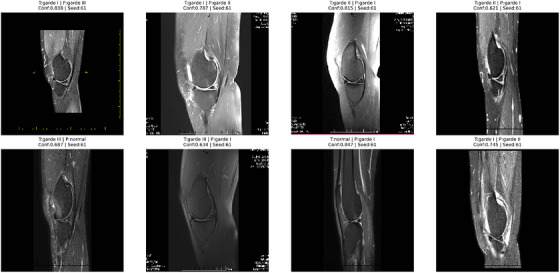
Representative misclassified MRI samples from the test set.
